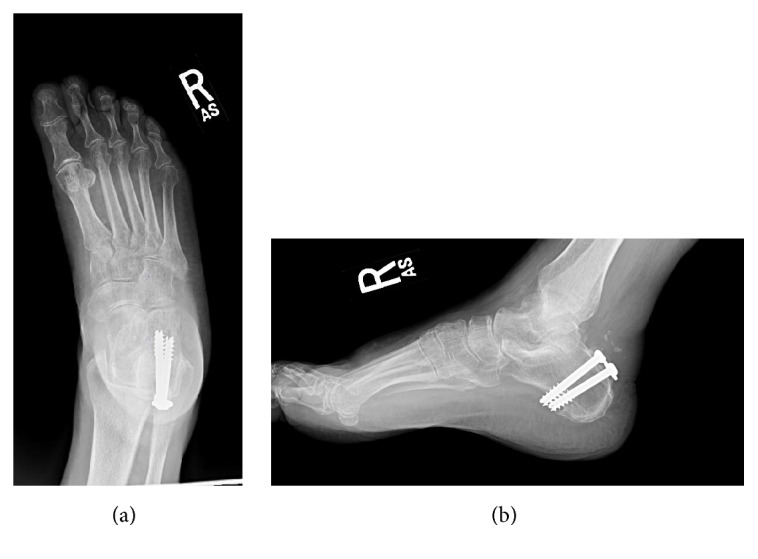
Anteroposterior (a) and lateral (b) radiographs showing complete healing of the tuberosity.
